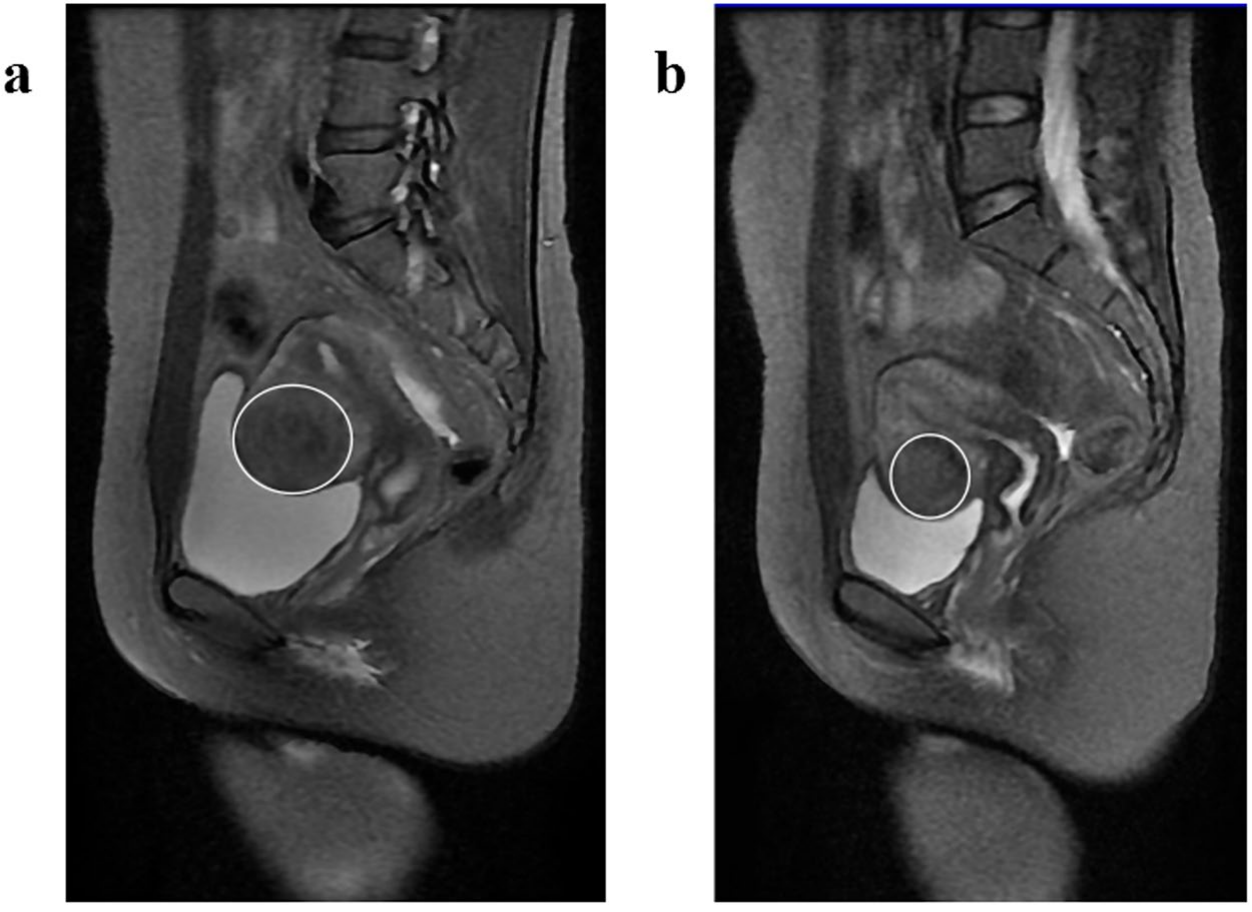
Magnetic resonance images showing the fibroid(white circles) before and after HIFU treatment. (a) before HIFU treatment, (b) three months after HIFU treatment.
